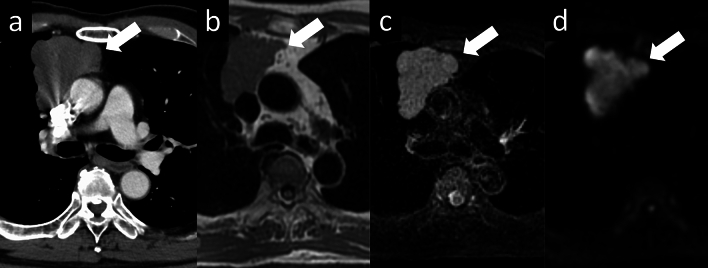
Thymoma (type B1). A 73-year-old man with type B1 thymoma. a Chest CT scan shows a 7-cm, lobulated, prevascular mass (arrow). b–d MRI shows high intensity on fat-suppressed T2WI and a slightly low ADC value (1.37 × 10^–3^ mm^2^/s) (arrow) (b: T1WI, c: fat-suppressed T2WI, d: DWI). CT, computed tomography; MRI, magnetic resonance imaging; T2WI, T2-weighted imaging; ADC, apparent diffusion coefficient; T1WI, T1-weighted imaging; DWI, diffusion-weighted imaging
